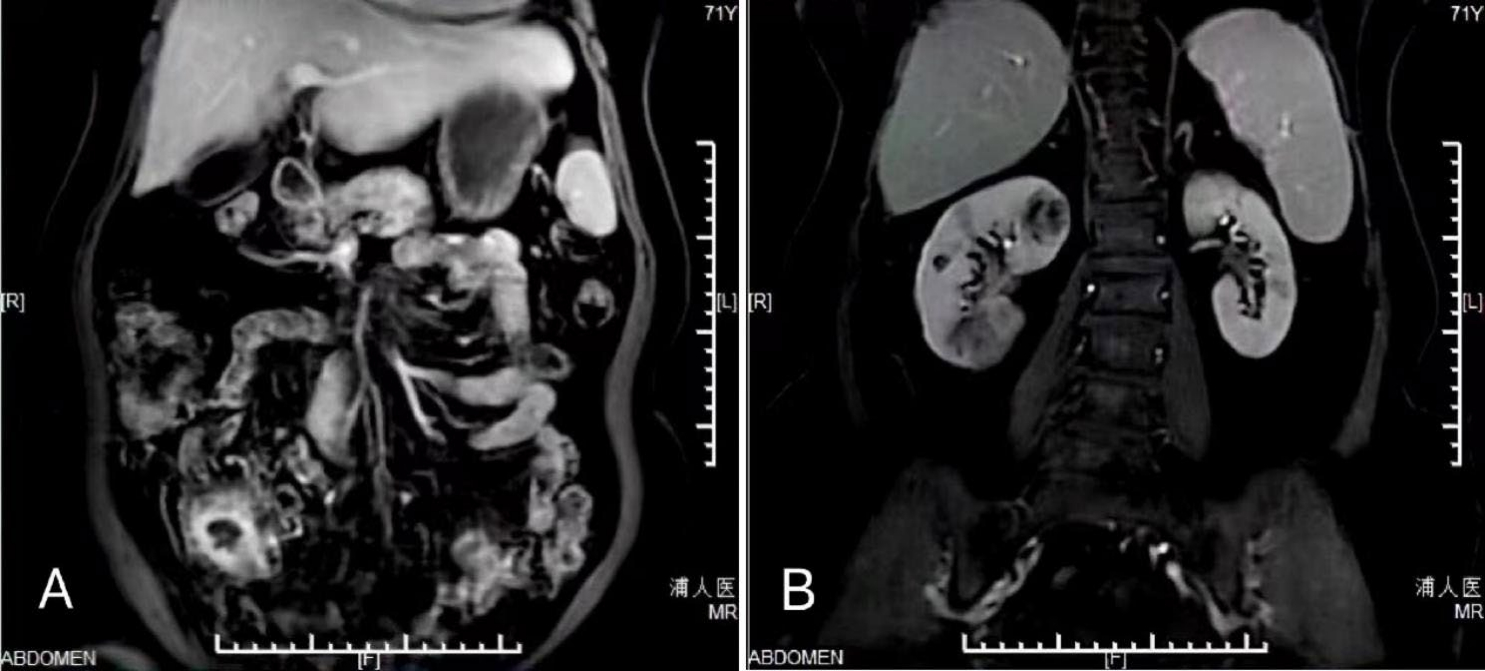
Magnetic resonance imaging show that the local small intestinal wall is irregularly thickened and intensifies in the right lower abdomen (A), with multiple hypersignal intensities observed in the right kidney (B)
